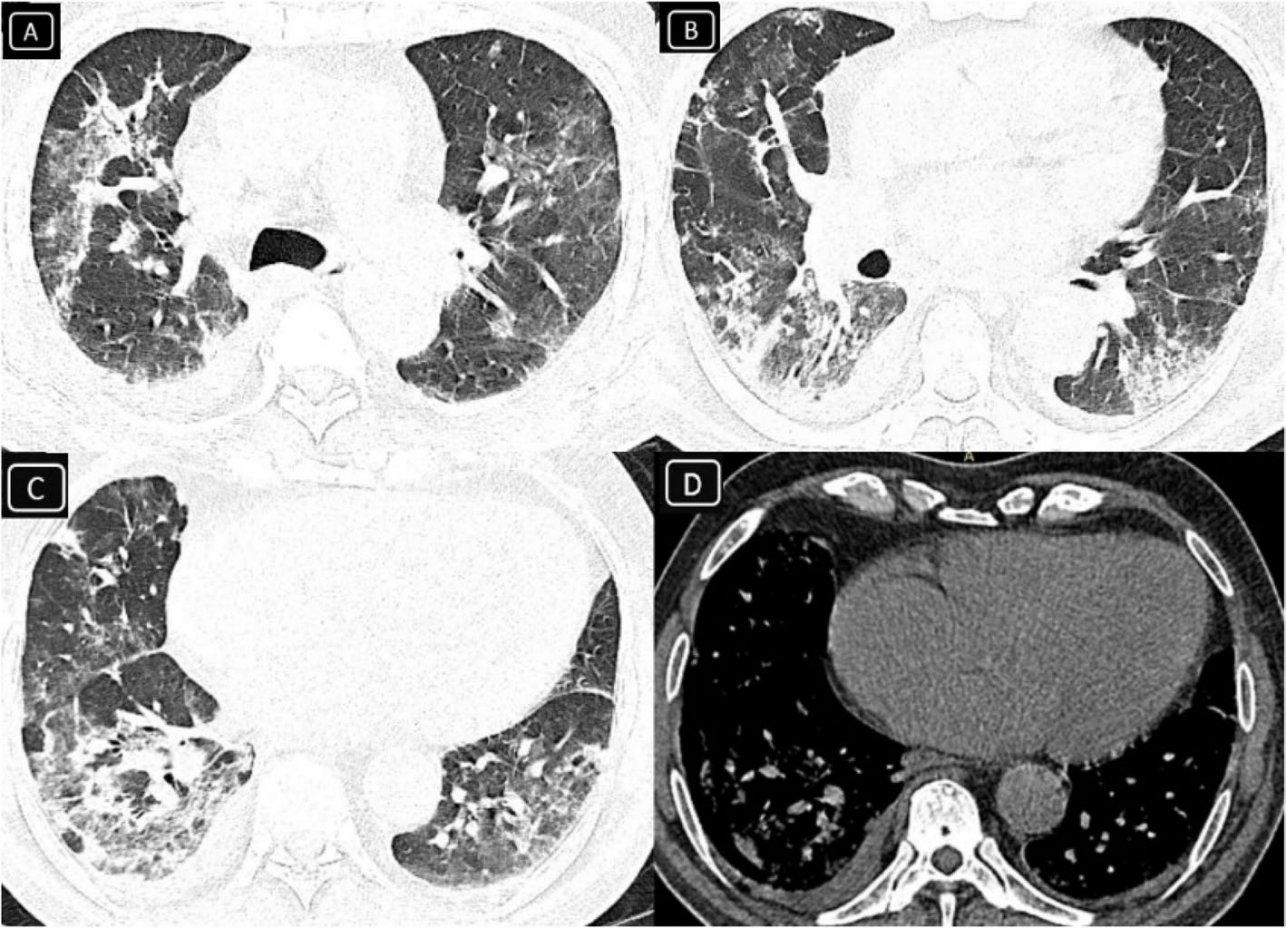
A–C Axial non-contrast chest CT (lung window) revealed bilateral widespread peripheral ground glass patches with septal thickening and peri-lobular fibrosis with architectural distortion (classic CT signs of severe COVID-19 infection). D Axial non-contrast chest CT (mediastinal window) also revealed mild right-sided and minimal left-sided pleural collection with cardiomegaly
